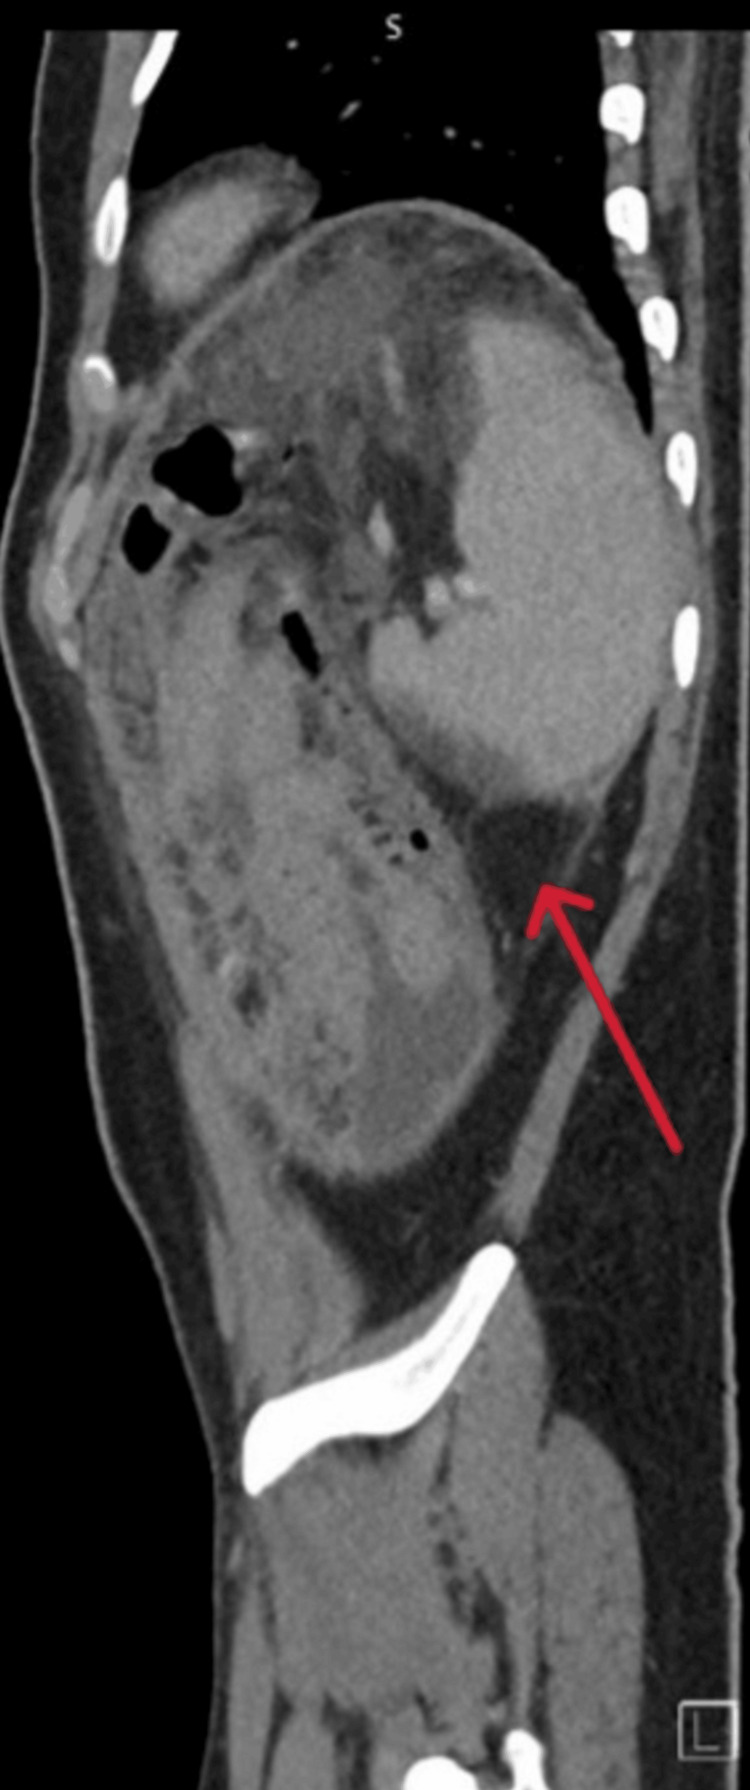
CT scan/sagittal view revealed pocket of fluid collection in the right pelvic area with septation and enhancing wall (red arrow)
